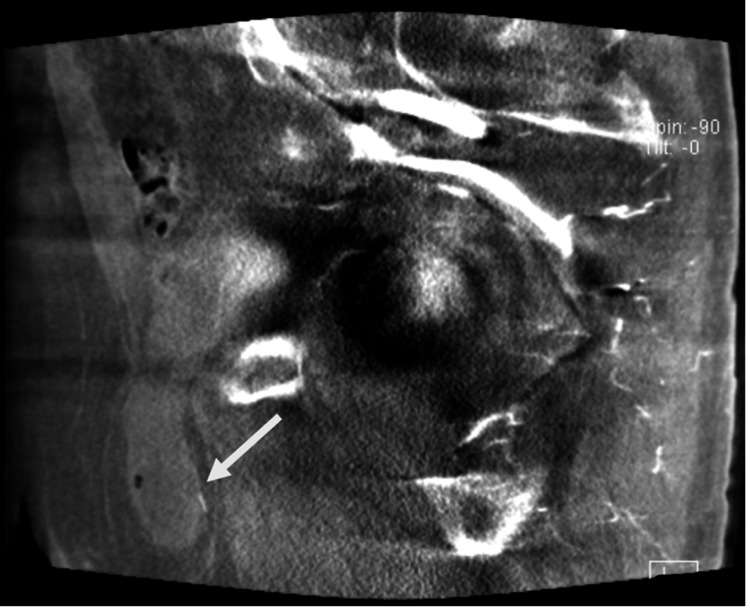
Sagittal reformats from an intraprocedural spin CT during prostate artery embolization show the bladder hernia extending into the inguinal canal CT: computed tomography
